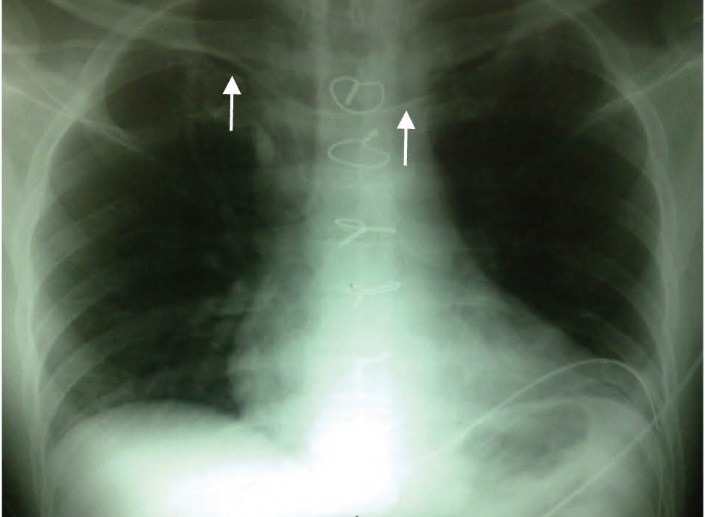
The overall malposition of the catheter tip on both sides was 5.7%.‏ In 5 patient, Pneumothorax was confirmed by chest x-ray. Pneumothorax occurred only in patients with right side catherization (4.3%). Subclavian arterial puncture occurred in 3 patients, 2 in right-sided attempts and 1 in left-sided attempt. The incidence rate was 1.6%.
